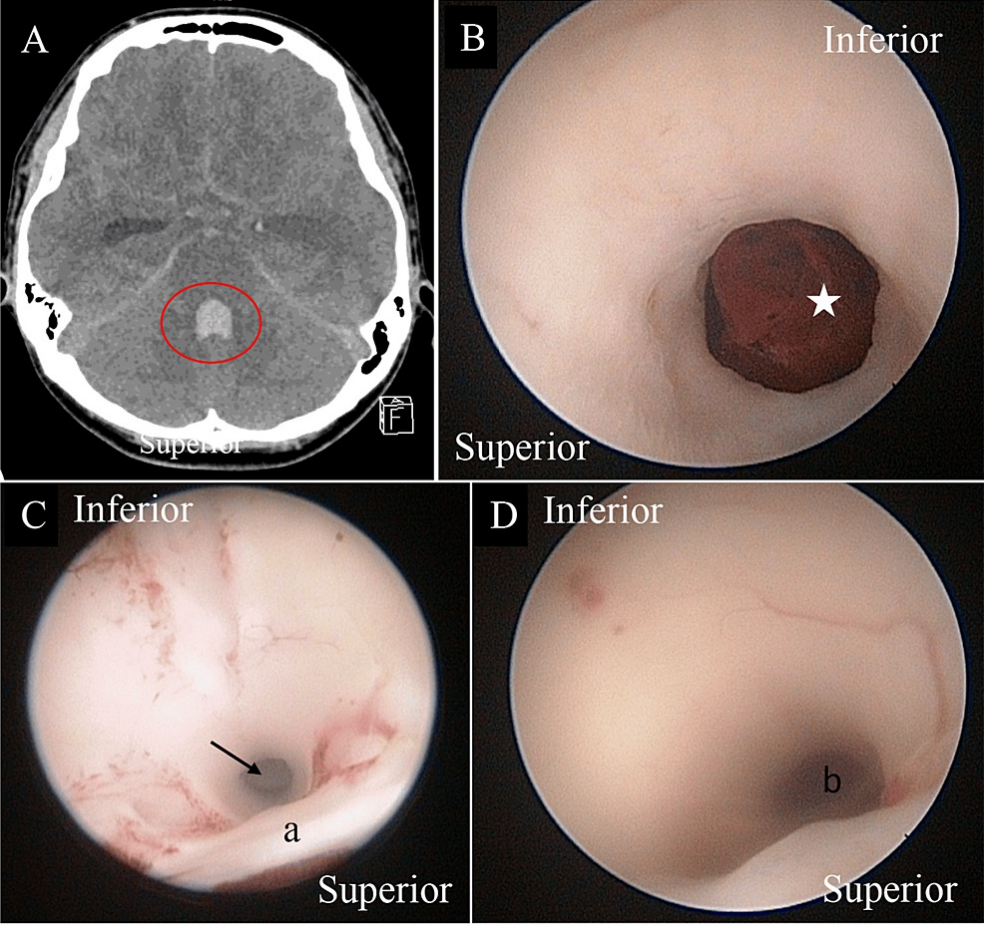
A child presented with sudden onset loss of consciousness with a Glasgow coma score of 6/15. (A) His CT brain disclosed intraventricular hemorrhages, particularly within the fourth ventricle (circle), secondary to rupture arteriovenous malformation. Images taken during the endoscopic washout of the hematomas. (B) Neuroendoscopy image at a closer look shows that the hematoma blocks the aqueduct of Sylvius (star). Ventricular irrigation was able to break down the clot, with (C) blood degradation products causing neuroinflammatory changes surrounding the ventricle. This was then removed successfully with the aqueduct re-establishment (arrow). (D) Another image from different patients shows the normal view of the aqueduct of Sylvius. a, posterior commissure; b, aqueduct of Sylvius.
